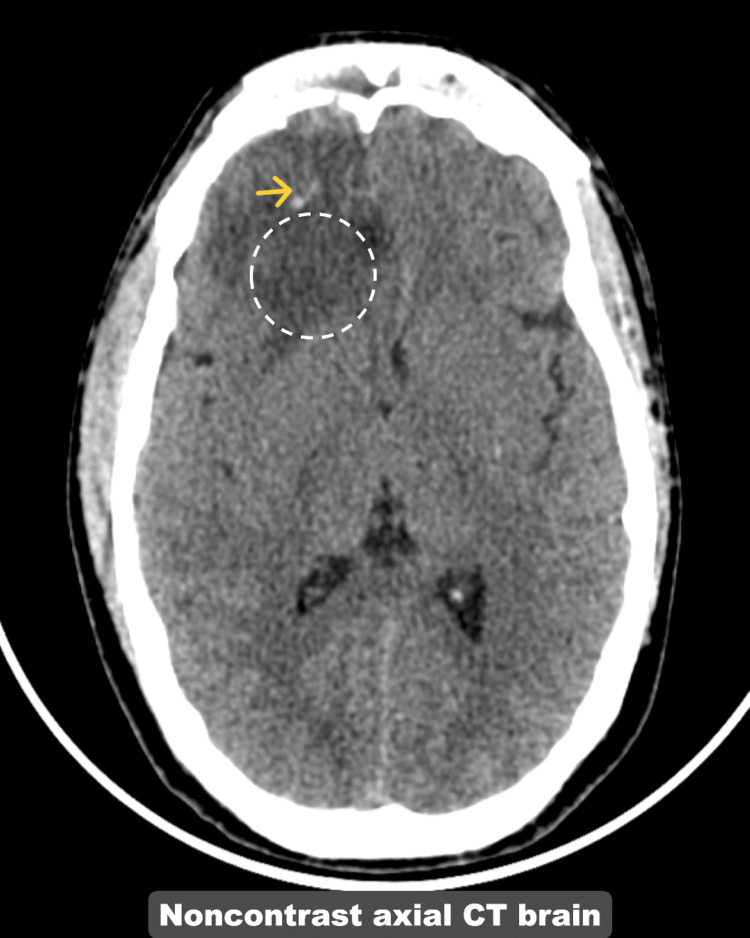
Day 2 non-contrast axial CT brain showing resolution of initial pneumocephalus Yellow arrow shows interval reduction in the previously noted hemorrhagic contusion in the right frontal region. White dotted circle shows complete resolution of the earlier identified intracranial air foci (pneumocephalus).
